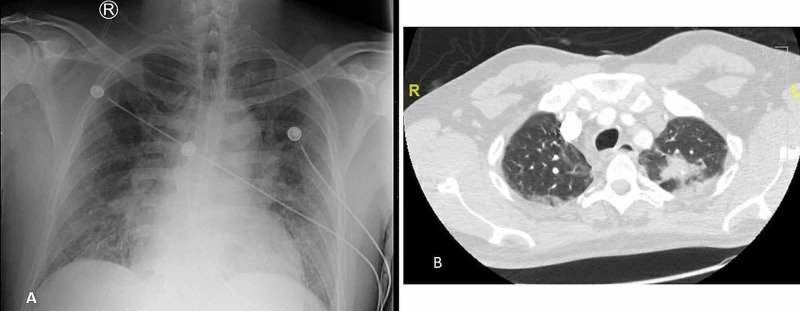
Chest X-ray, AP view showing a hazy right middle lobe opacity and bibasilar linear opacities (A). CT angiography revealing patchy consolidations on bilateral lung apices; more prominent on the left side than the right (B). AP, anteroposterior
